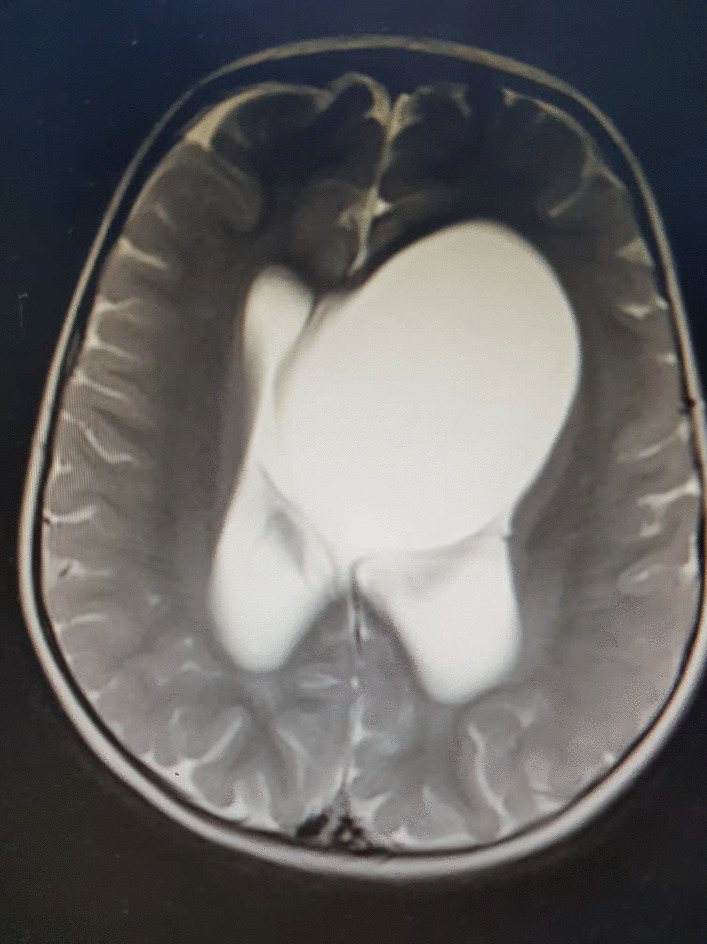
Cranial MRI imaging demonstrated a large left-hemispheric cystic process with a midline shift, well-defined thin-walled suprasellar arachnoid cyst measuring 3 × 5 × 7 cm causing hydrocephalus ventriculomegaly
